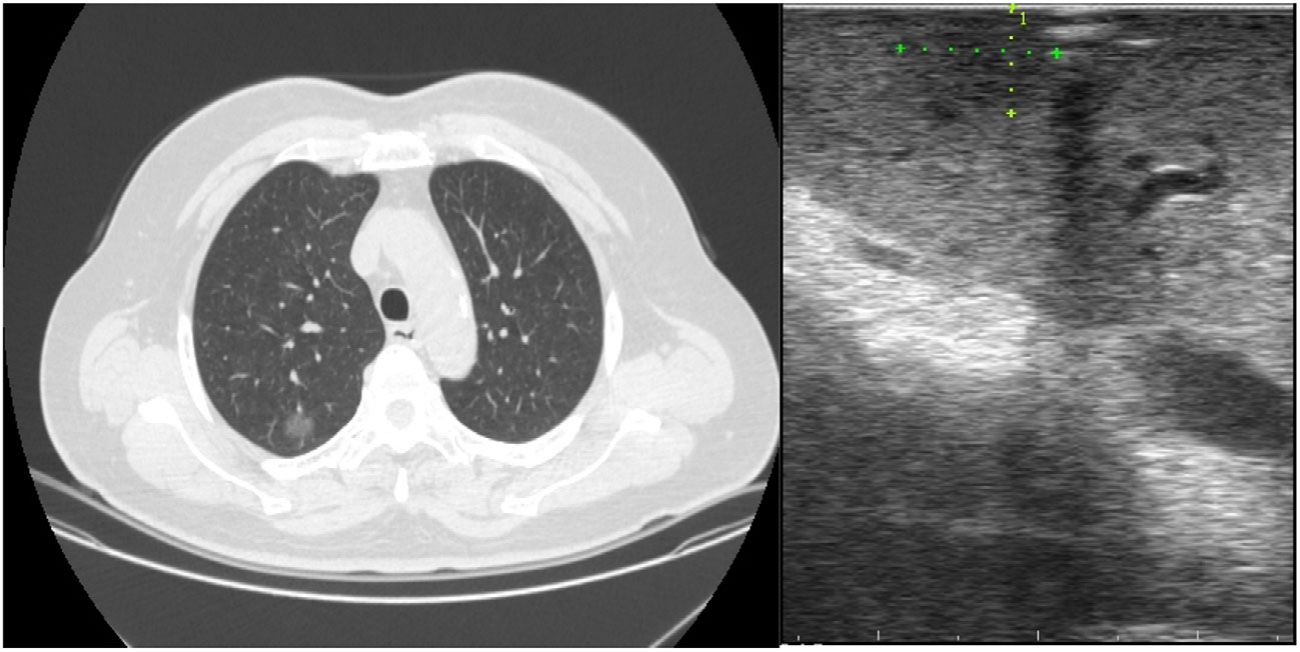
(a) CT scan and (b) ultrasound which confirmed the presence of pure ground‐glass nodules.
